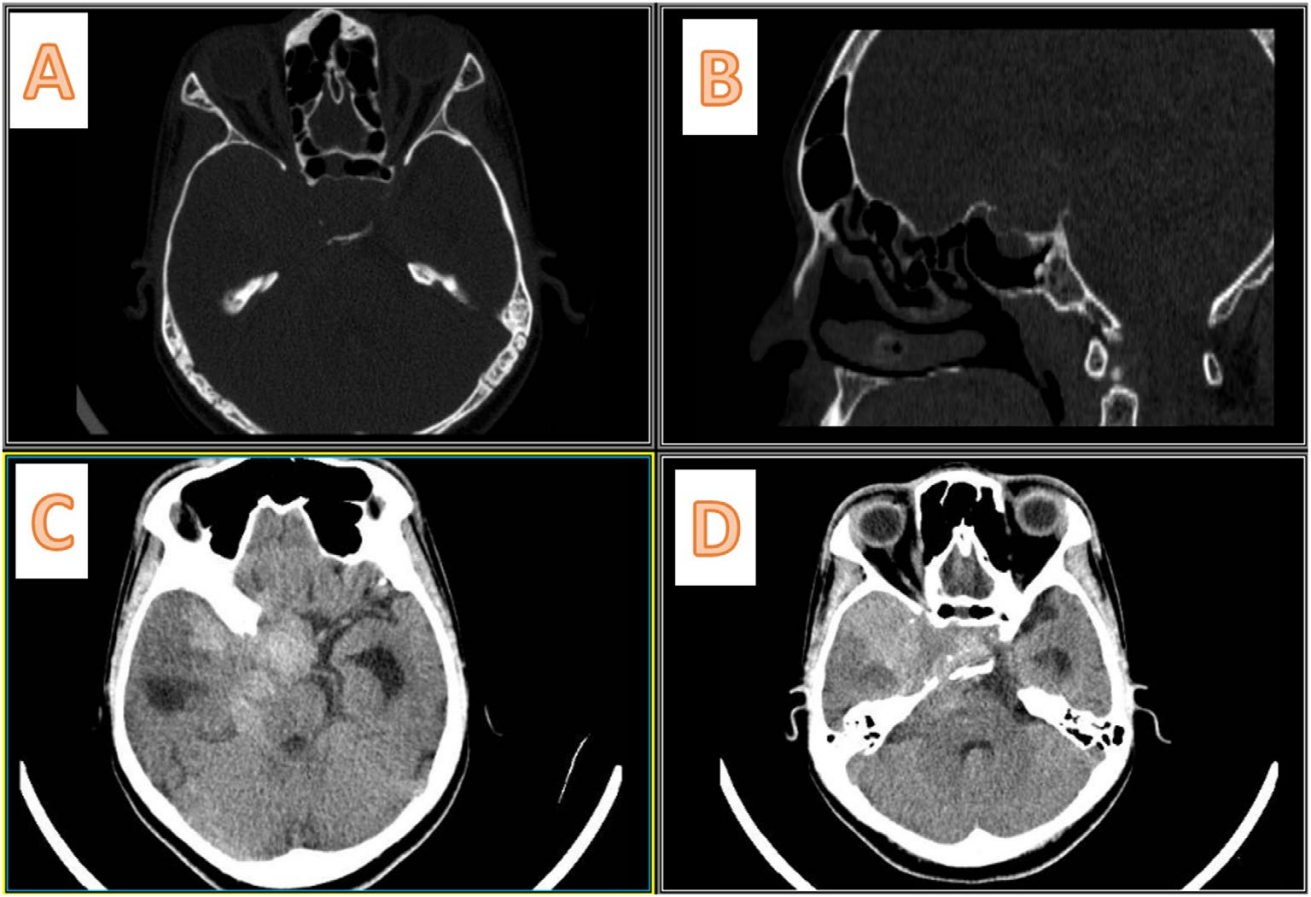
Preoperative non‐contrast enhanced brain CT scan. (A, B) The bone window; there is a widening of the sella turcica in A and Erosion of the sella floor in sagittal view in (B). (C, D) The parenchymal view of the brain; there is a heterogeneous hyperdense lesion in the sella turcica with extension to the right middle fossa and posterior fossa through the tentorium cerebelli. There are enlarged temporal horns of the lateral ventricles bilaterally, which indicate hydrocephalus.
